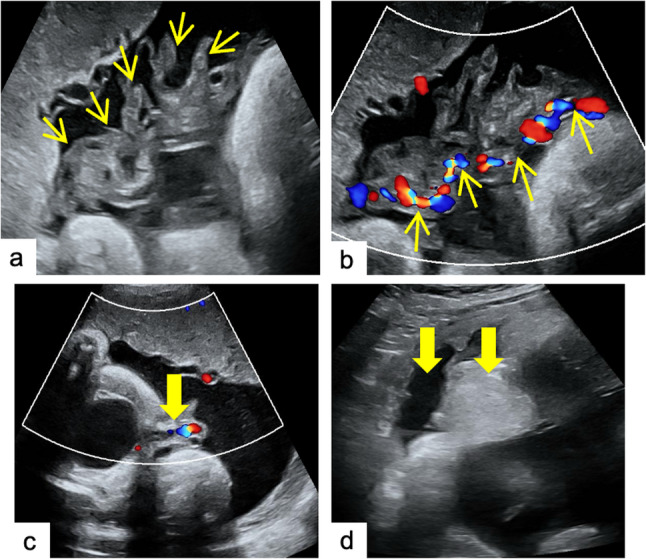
Prenatal ultrasound and Color Doppler imaging (CDI) findings. a Disorganization of the umbilical cord structure, with flocculent hypoechoic and mildly echogenic material floating in the surrounding amniotic fluid (arrows). b CDI demonstrates attenuated umbilical vessels and surrounding avascular flocculent zones (arrows). c The cord insertion site shows a thinned umbilical artery and a wrinkled, discontinuous amniotic membrane with weak internal echoes (arrow). d A large anechoic area between the placenta and uterine wall, containing flocculent material (arrows)
